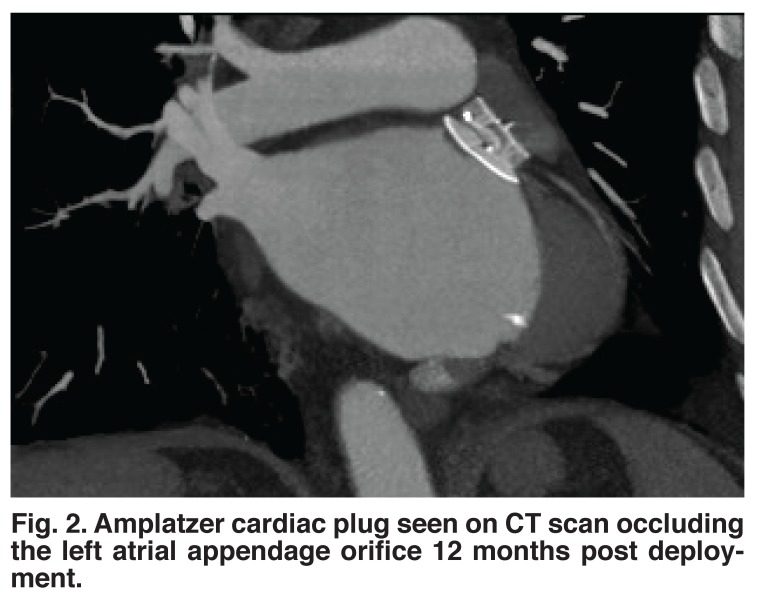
Amplatzer cardiac plug seen on CT scan occluding the left atrial appendage orifice 12 months post deployment.
